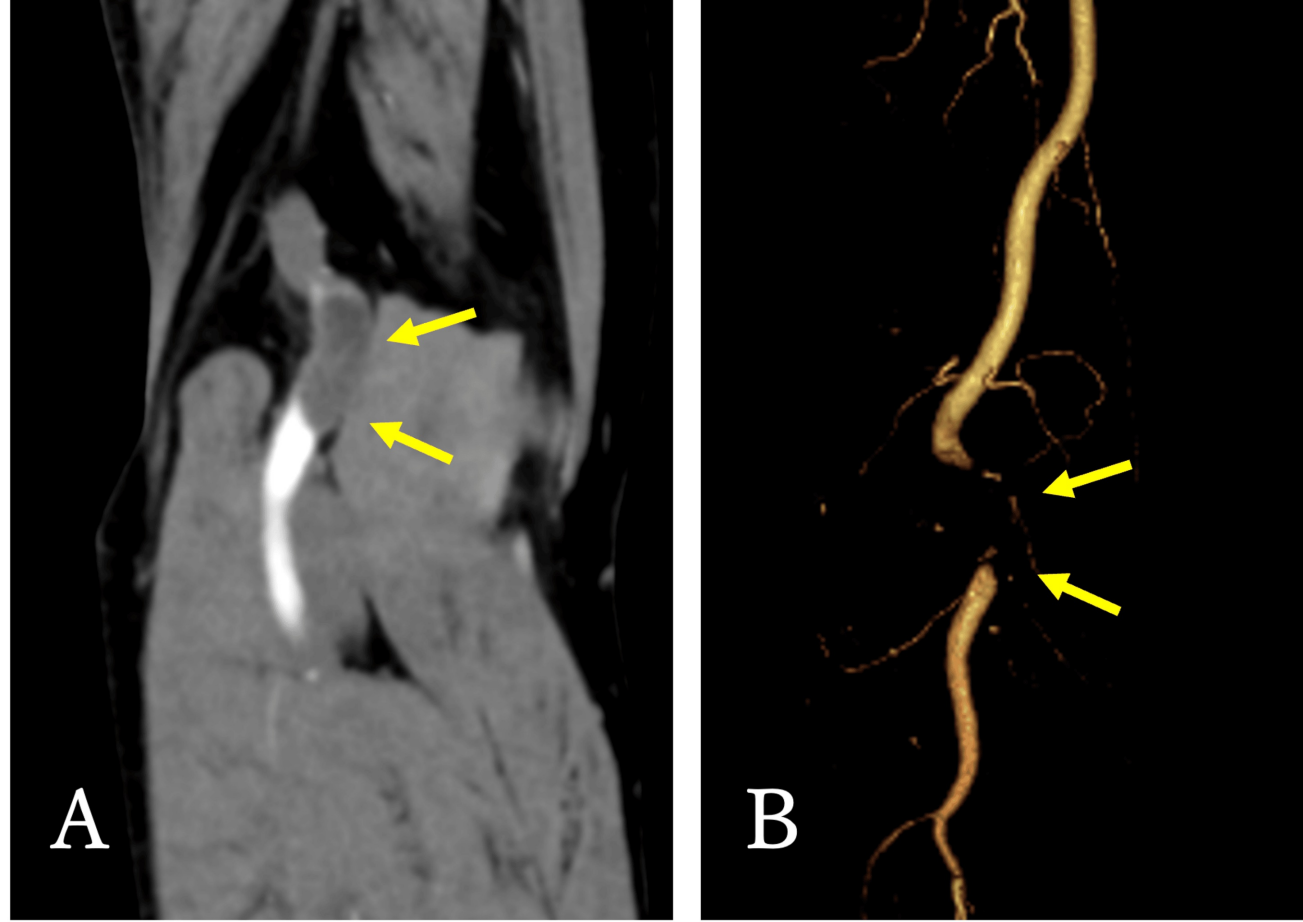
Contrast-enhanced computed tomography. (A) A low-density area was apparent in the right popliteal fossa (arrows). (B) The popliteal artery was almost occluded (arrows).
